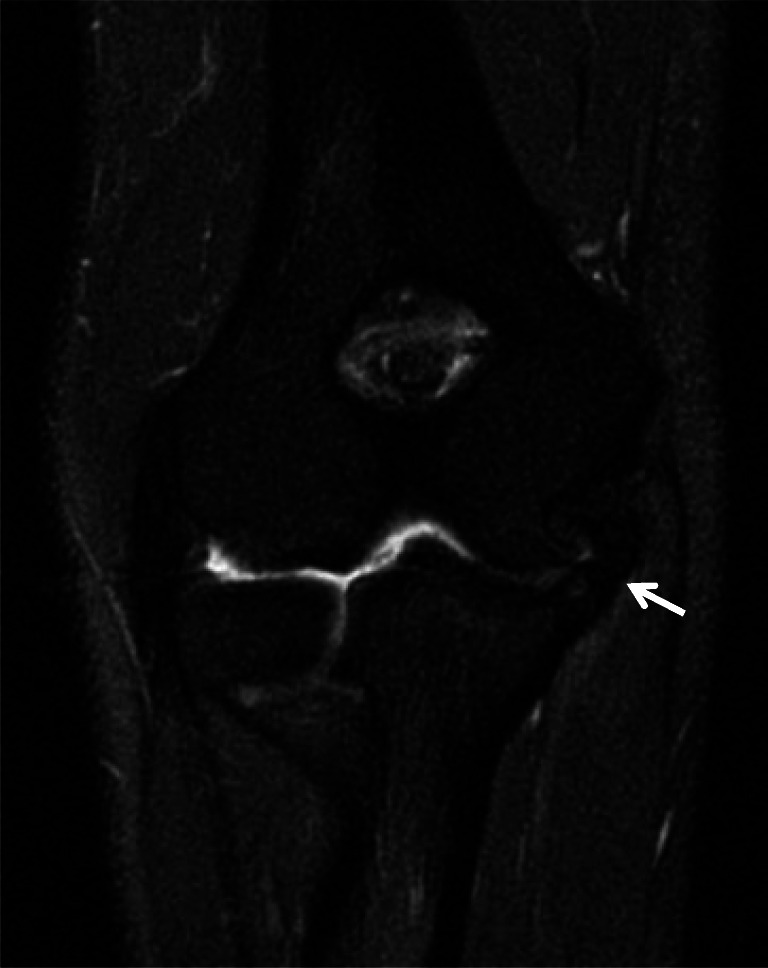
Coronal T2 MR image of the elbow demonstrates chronic low signal thickening of the UCL (arrow)
